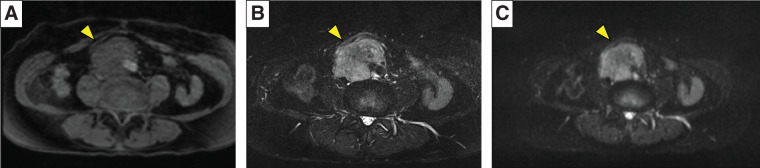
Magnetic resonance images showing a tumor with low intensity on T1-weighted images (A, yellow arrowhead), and high intensity on T2-weighted and diffusion-weighted images (B and C, yellow arrowheads).
